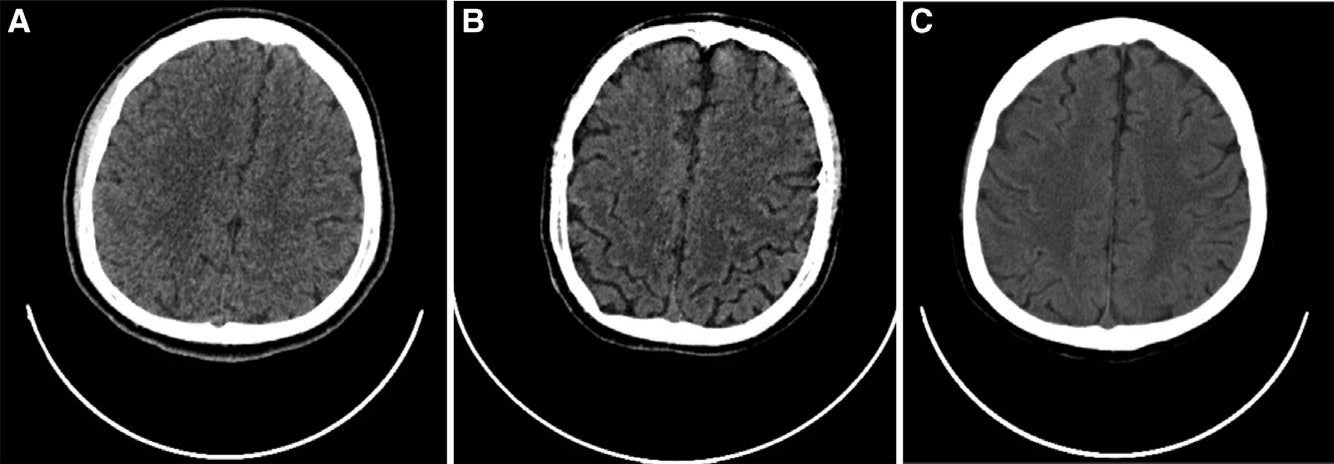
(A) Head CT on admission showed no significant abnormality. (B) Head CT examination after 2 weeks in hospital showed no obvious abnormality. (C) Head CT examination 5 weeks after hospitalization revealed mild cerebral atrophy. CT = computed tomography.
